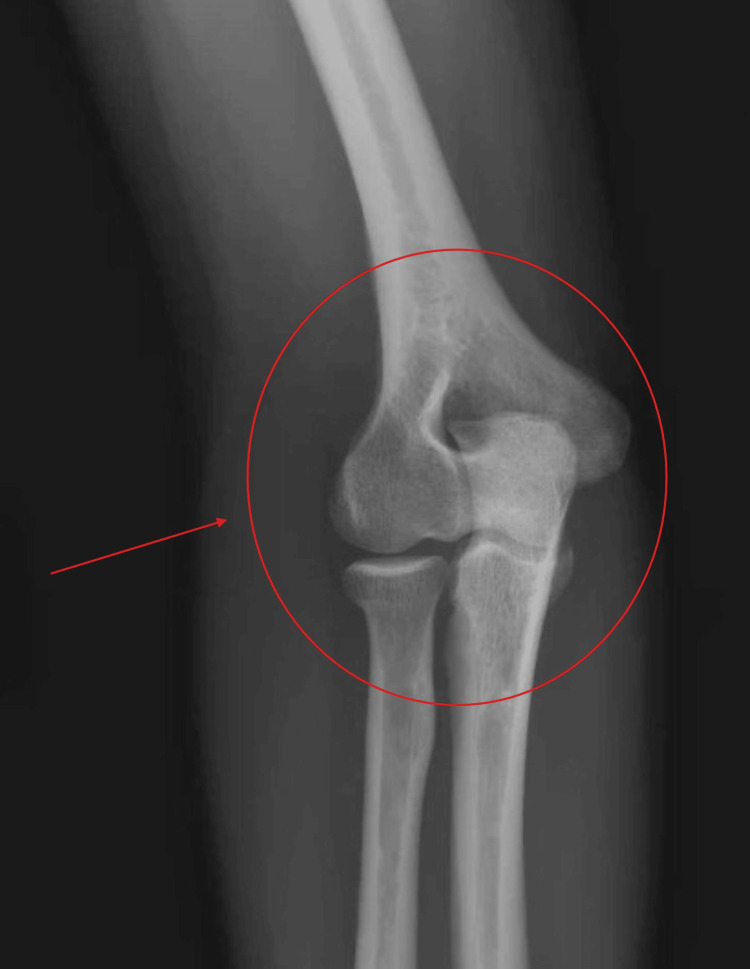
Anteroposterior radiograph of the right elbow showing normal osseous alignment The highlighted section demonstrates normal osseous alignment of the bone.
